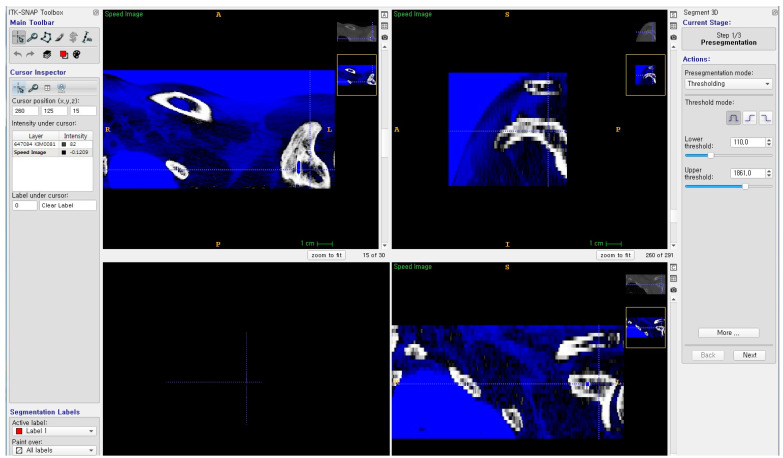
ITK snap (ver 3.4.0) was used for reconstructing CT axial images to a 3D structure image.
